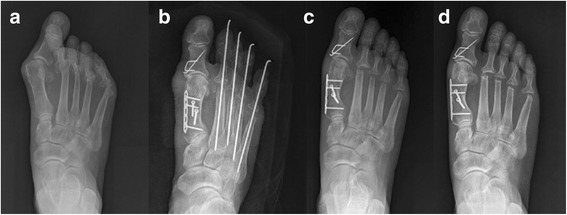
A case with plate augmentation: a A preoperative radiograph of a 61-year-old woman with rheumatoid arthritis. b A postoperative radiograph taken immediately after surgery. c The 1–2 inter-metatarsal angle and altered margin of lateral cortex changed by less than 5°. d Radiograph taken at 24 months following surgery
